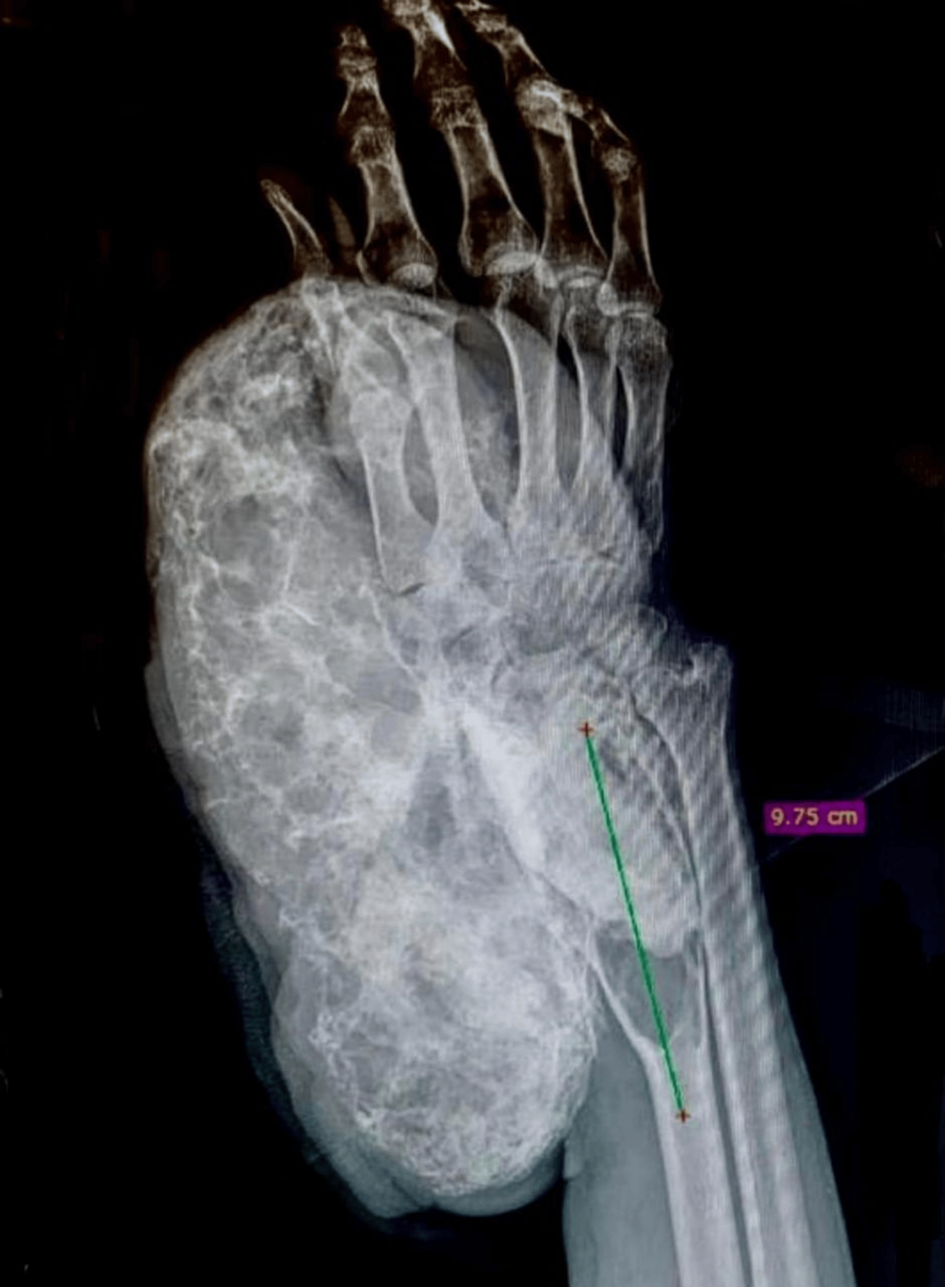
Radiograph of the right forearm in anteroposterior projection with an expansive bone lesion that involves the distal radius
